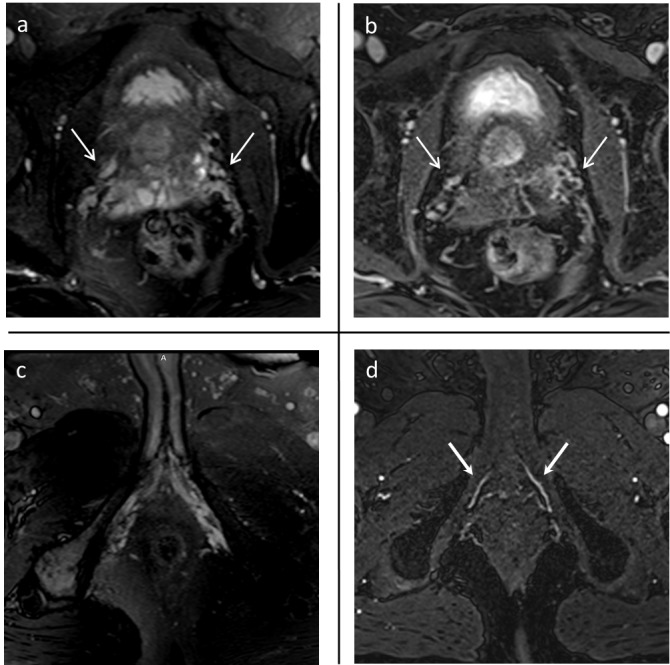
MR images in a 67-year-old man with prostate carcinoma (Case 6) Axial true-FISP (a) and T1-weighted VIBE contrast-enhanced images (b) show neurovascular bundles (NVBs) (arrows). The accessory pudendal arteries (APAs) are not visible on axial true-FISP (c) but brightly enhance on T1-weighted VIBE contrast-enhanced images (d) (arrows). Axial true-FISP (a, c) are non-contrast-enhanced images. The T1-weighted VIBE contrast-enhanced image (b) is the venous phase. The T1-weighted VIBE contrast-enhanced image (d) is the arterial phase after 30 seconds of dynamic. In axial true-FISP and T1-weighted VIBE contrast-enhanced images, the depiction of NVB was good, but the depiction of APA was superior in the T1-weighted VIBE contrast-enhanced image.
